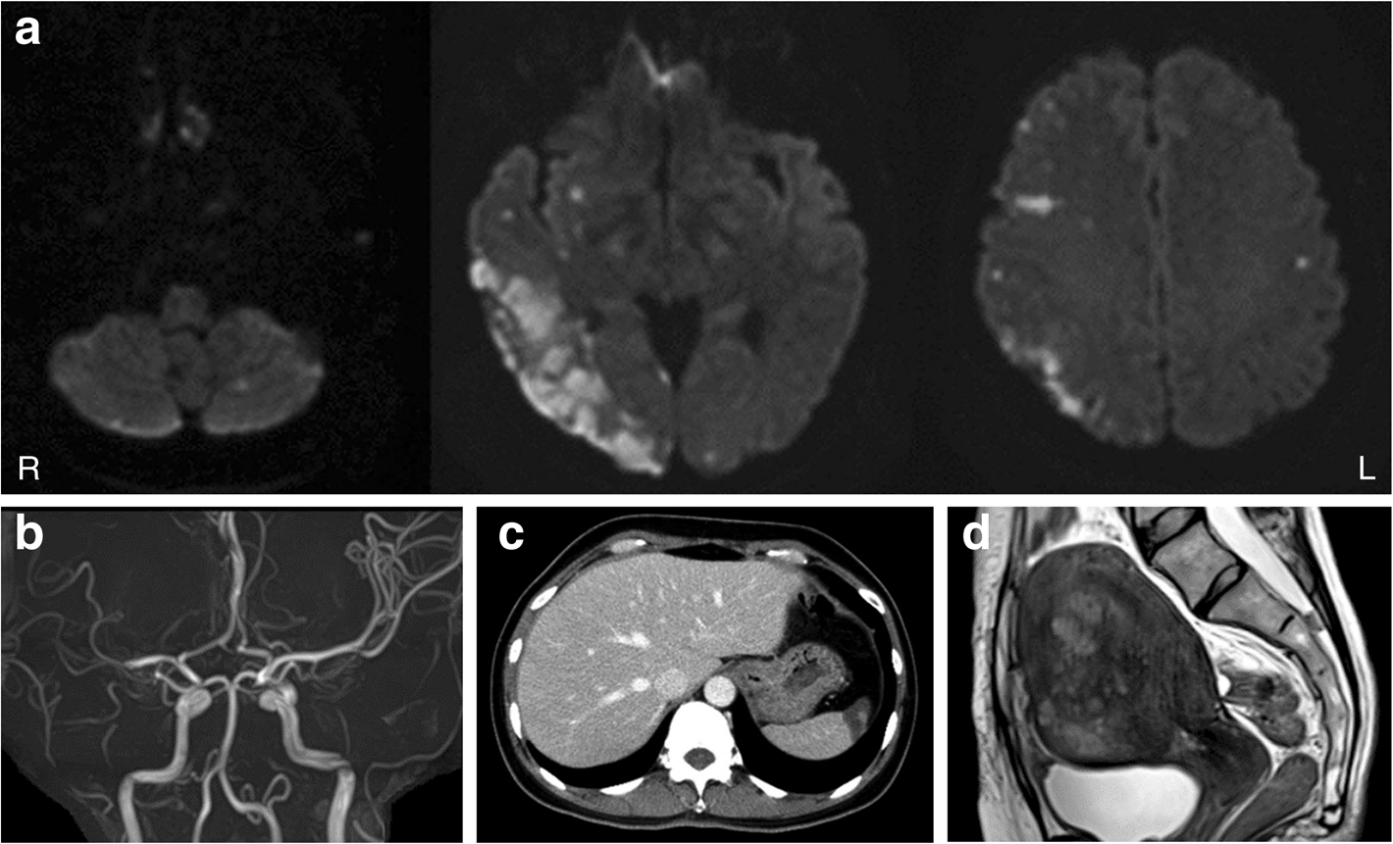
Diffusion-weighted magnetic resonance imaging (MRI) scan of the brain reveals multiple infarctions in the cerebellum and cerebrum (a). The M2, M3, and M4 portions of the right middle cerebral artery were not well visualized by MR angiography (b). Contrast computed tomography revealed splenic infarction (c). Giant adenomyosis was revealed by a T2-weighted MRI scan of the pelvis (d)
